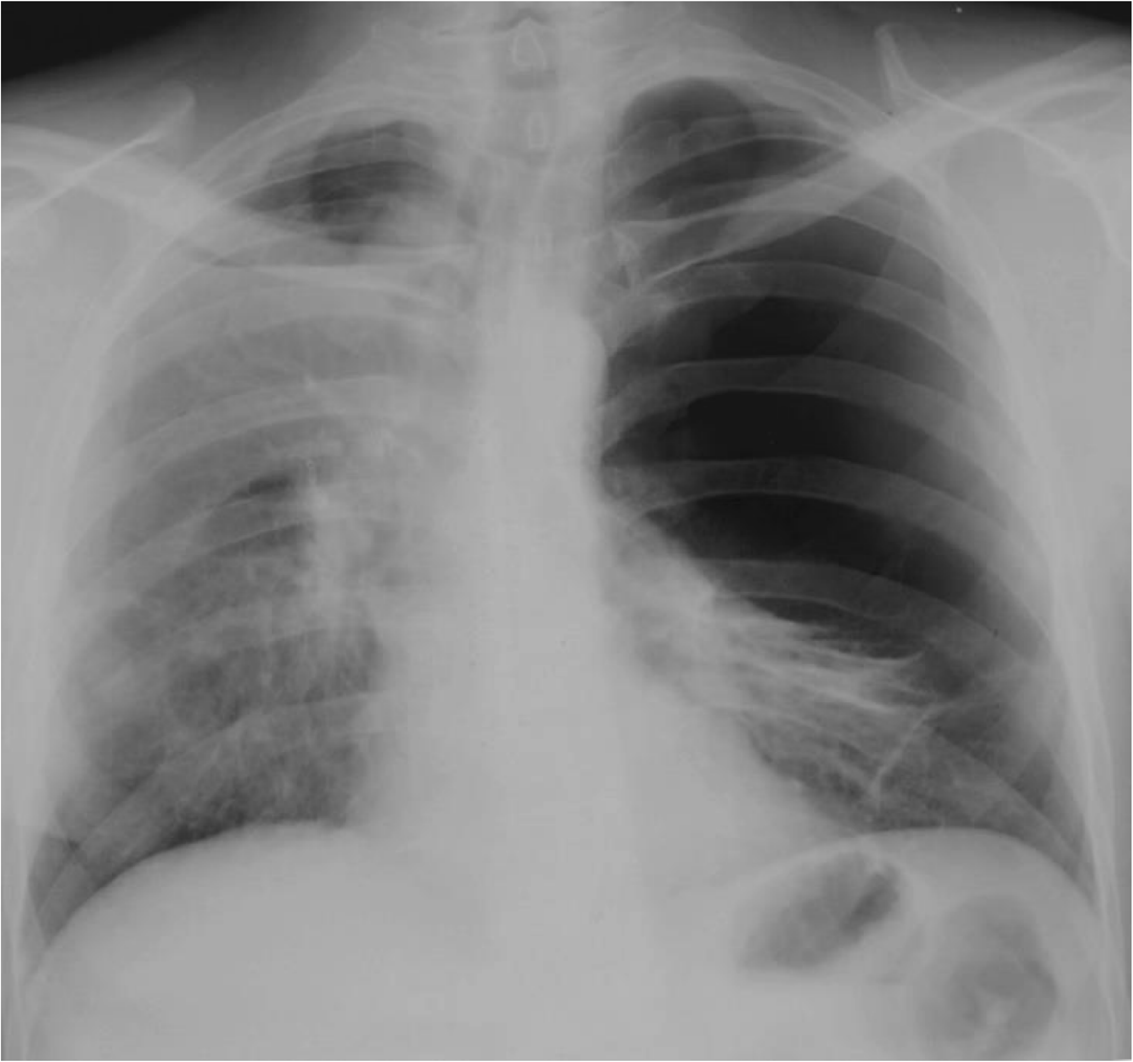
Chest plain film past the first surgery. Giant bull on the right is enlarged and fills about 2/3 of hemithorax volume. Mediastinum is shifted to the right. Right lung is not extended
